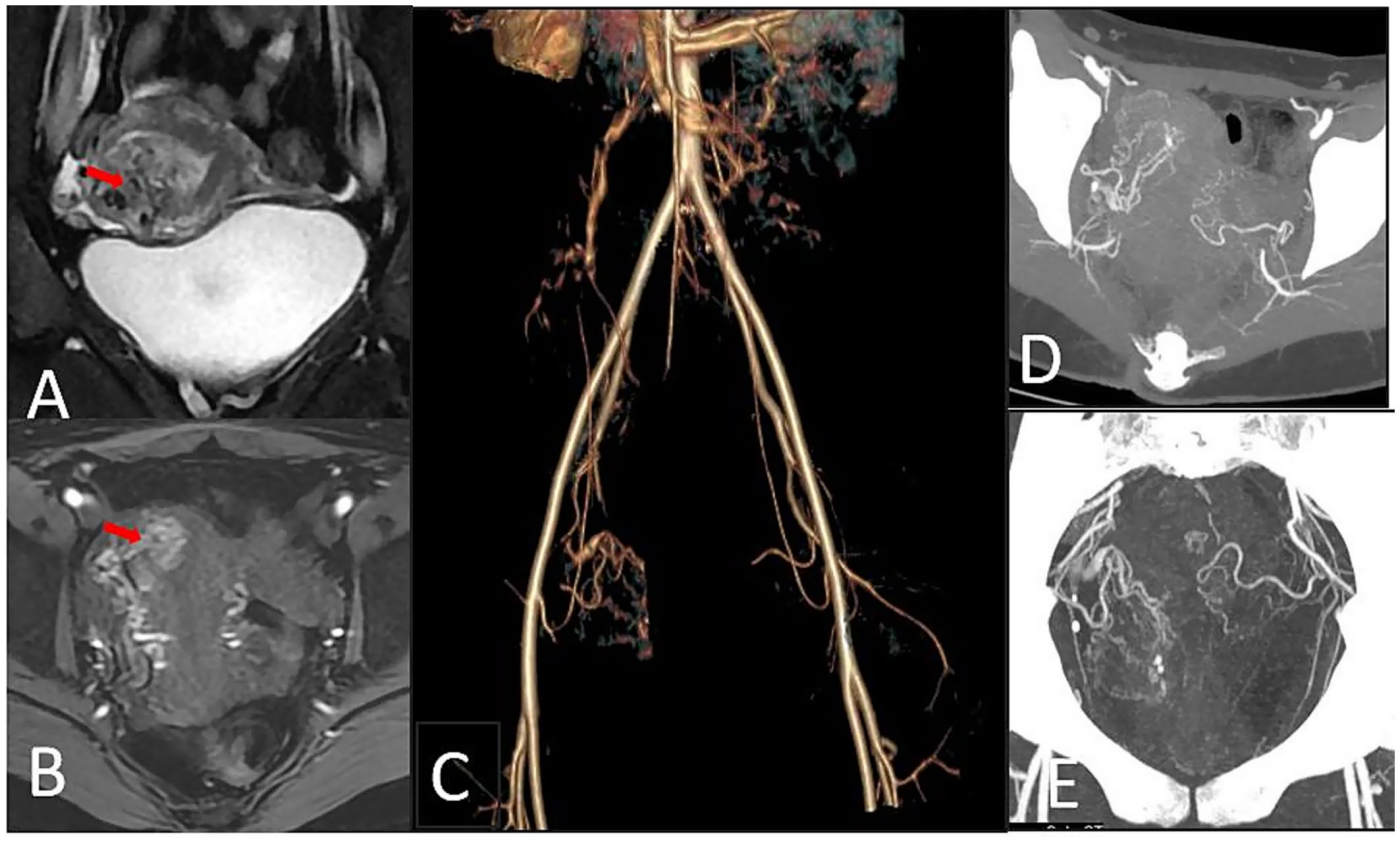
MRI and CTA images of two patients before drug treatment. (A,B) Coronal and dynamic scan images of Patient 1, which show early arterial development and a significant increase in the number of uterine veins on the right wall. (C–E) Postprocessed CTA images of Patient 2, which indicate that the right uterine vein appears earlier and significantly increases in size compared with the left.
